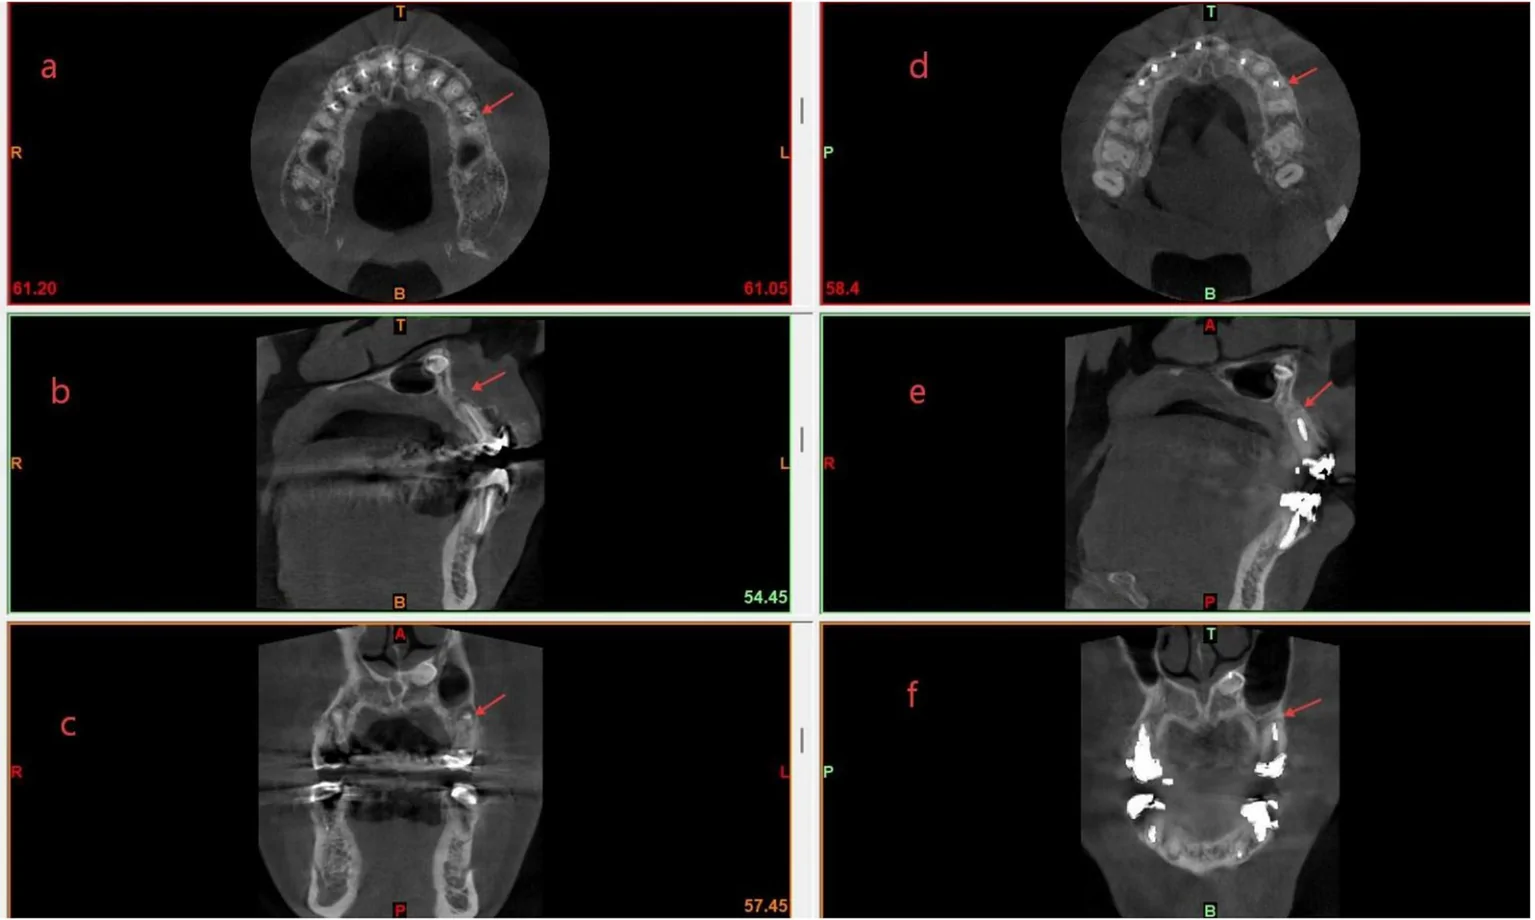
The red arrow indicates the lesioned area and the surgically healed area. CBCT images at T0 and T1 were imported into Mimics software and analyzed from three perspectives (in panels a, d; panels b, e; panels c, f). The three-dimensional imaging clearly demonstrates complete healing of the apical bone graft material and autologous bone tissue.
